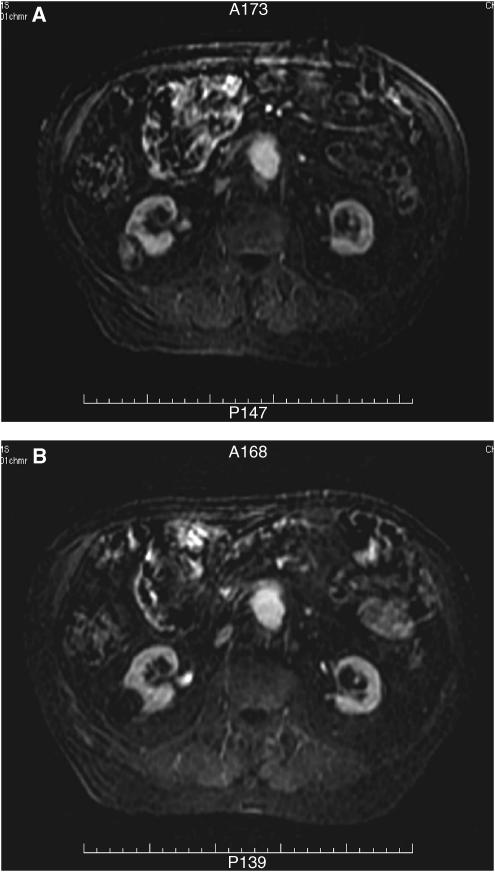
Subtraction MRI films, taking the FAME precontrast series from the 1 min postcontrast images. Patient with a right primary renal tumour (A) before HIFU showing contrast uptake within the target tumour, and (B) 12 days after HIFU, showing no contrast uptake within the target tumour, consistent with ablation.
